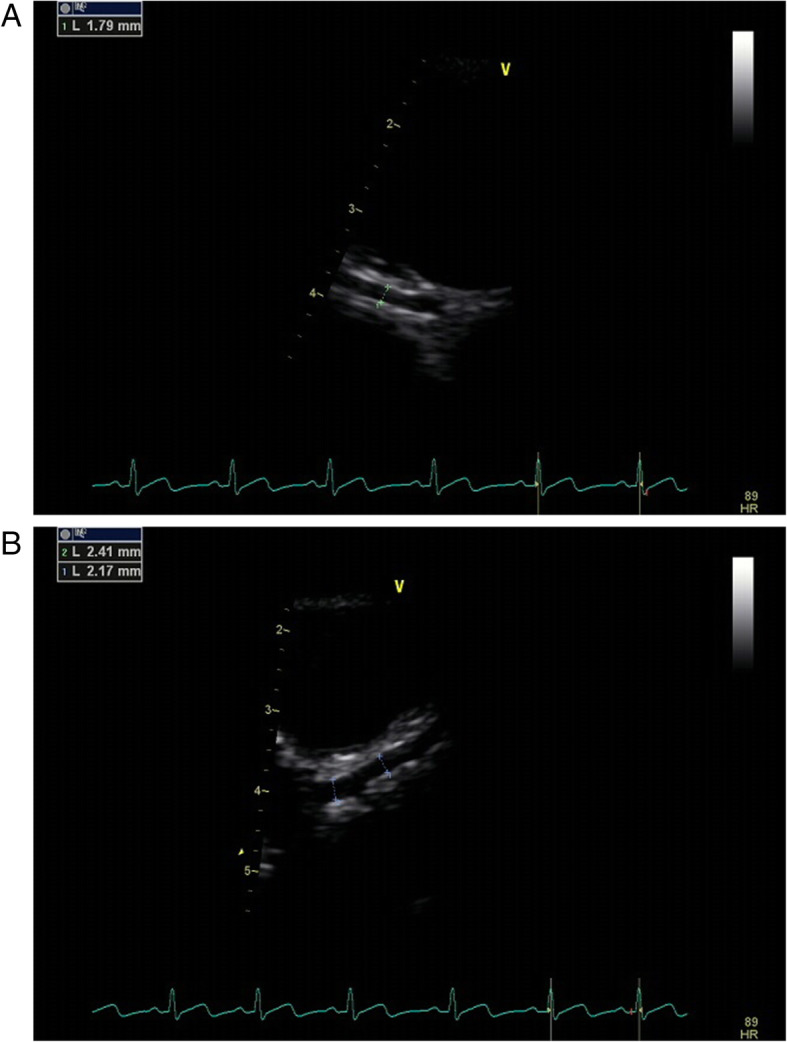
Short-axis echocardiographic view used for coronary artery diameter measurements. A RCA, right coronary artery; B LCA, left coronary artery; LAD, left anterior descending coronary artery
